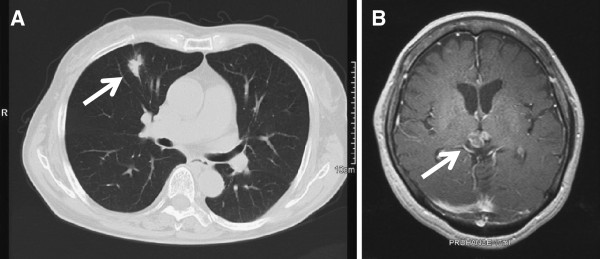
Chest computed tomography scan and magnetic resonance imaging scan of the brain after the treatment. (A) Chest computed tomography scan after the chemotherapy revealed shrinkage of the primary lesion (arrow), which was evaluated as a partial response. (B) An axial T1-weighted magnetic resonance imaging with gadolinium injection after the stereotactic radiation and chemotherapy showing resolution of hydrocephalus, and shrinkage of the pineal region tumor (arrow).
